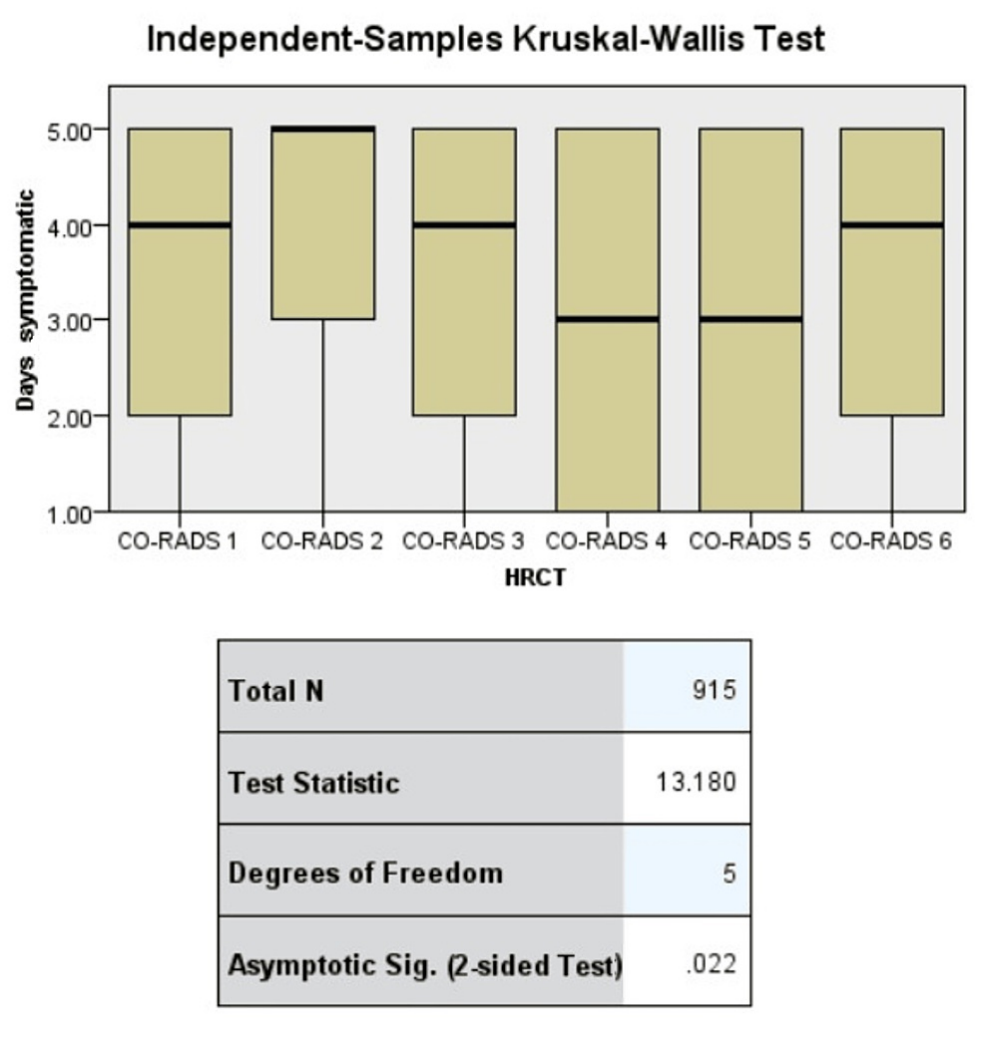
Symptoms of COVID-19 disease. COVID-19: coronavirus disease 2019; HRCT: high-resolution computed tomography; CO-RADS: coronavirus disease 2019 reporting and data system
